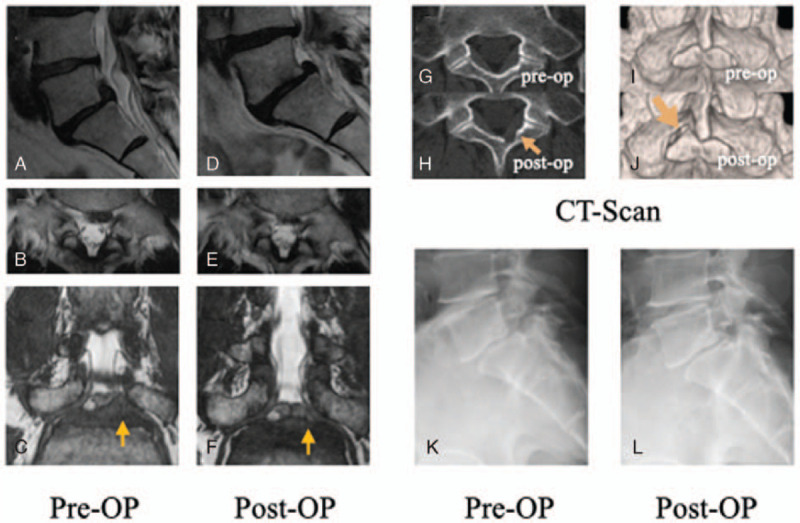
A 55-year-old man who had been suffering from radiculopathy of the L5 area with spondylolisthesis underwent a full endoscopic discectomy via the interlaminar space (FESS-IL) for L5/1. At 1 year after surgery, there was no sign of recurrence, herniation, or progression of slippage (K and L). Preoperative sagittal (A) axial (B) and coronal (C) images on T2-weighted magnetic resonance imaging (MRI), and axial and 3D image on computed tomography (CT) (G and I). Postoperative (1 year) sagittal (D) axial (E) and coronal (F) images on T2-weighted MRI. Axial and 3D-CT image on CT (H and J) shows only a small amount of drilling was performed on the inferior border of the lamina.
